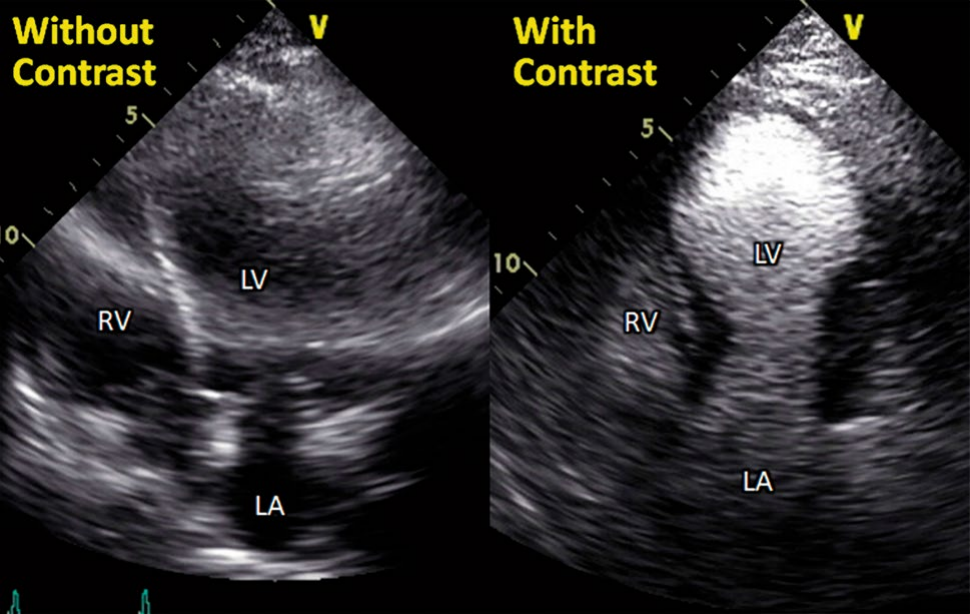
Opacification of wall motion abnormalities by contrast echocardiography. Left and right panels show apical four-chamber views with and without contrast agent in a case of takotsubo syndrome. Contrast echocardiography helps opacification of wall motion abnormalities, especially in patients with poor echo images. LA, left atrium; LV, left ventricle; RV, right ventricle
